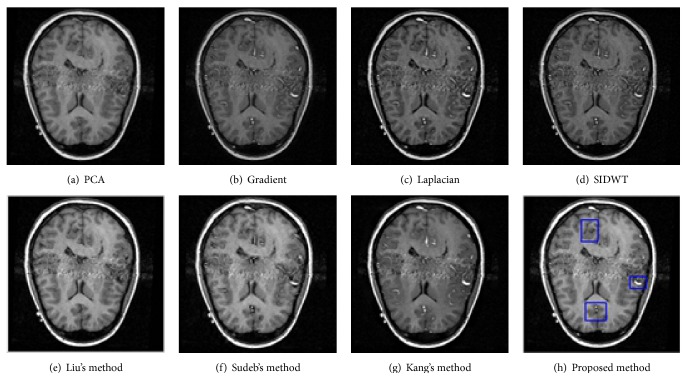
The fusion results of MRA and MRI.
